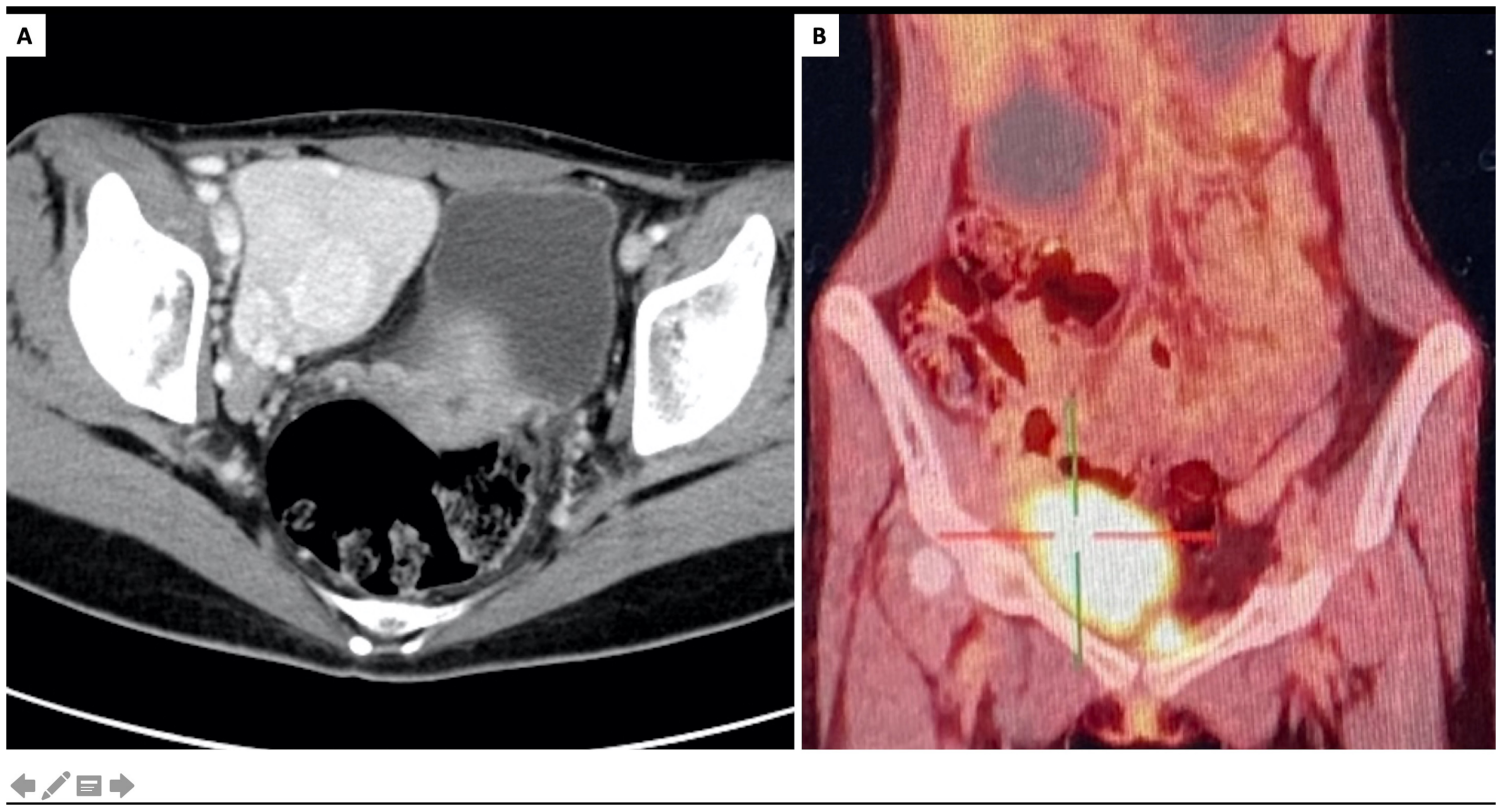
Characterization of the pelvic mass. (A) Contrast-enhanced CT, axial view showed a pelvic tumor at right iliac fossa with marked enhancement and hypertrophied vessels. (B) PET/CT scan, coronary view showed increased metabolic activity of the pelvic tumor.
